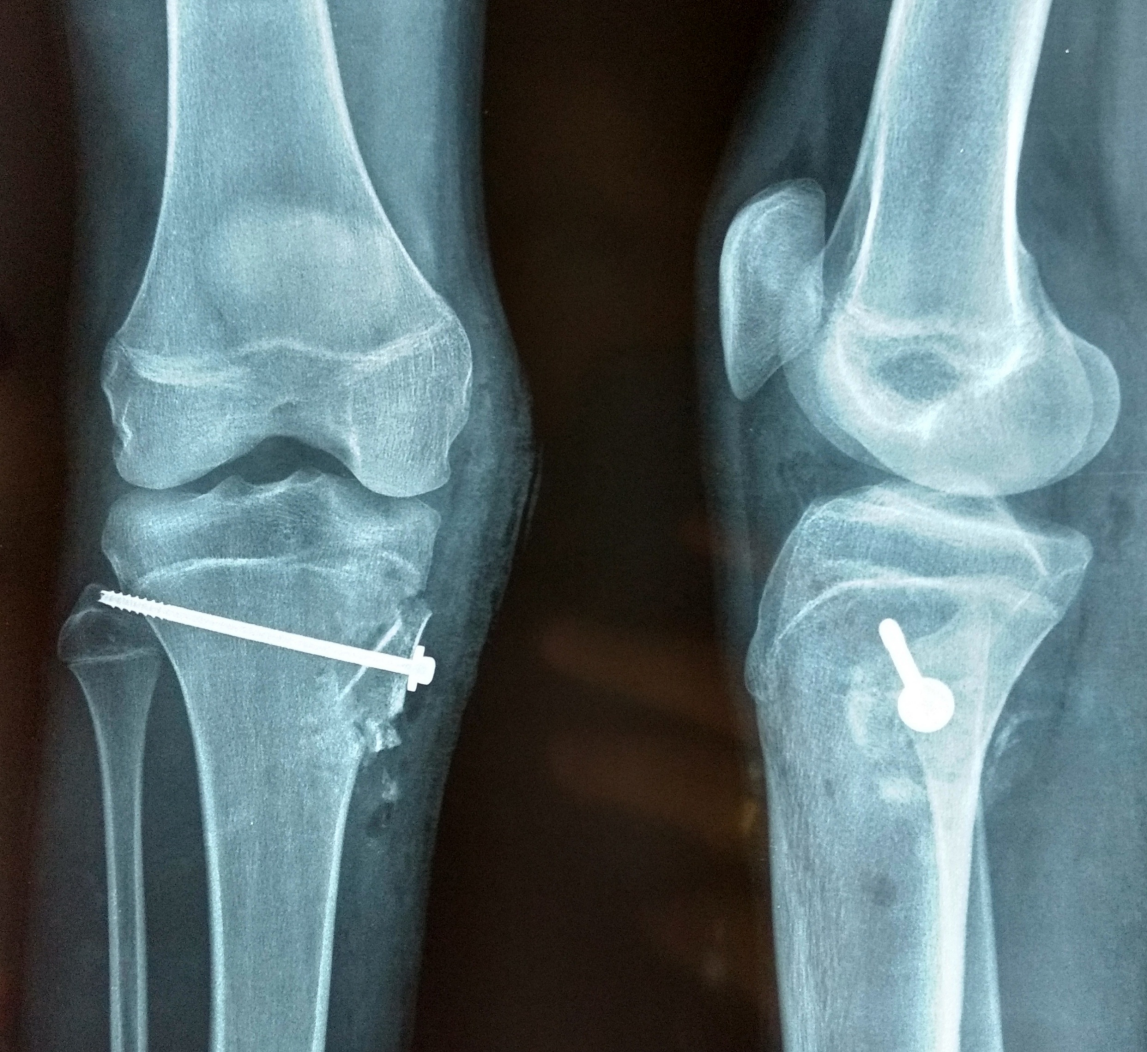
Postoperative radiographs The images show excision of the lesion and the cavity filled with bone graft and fixed with a screw.
